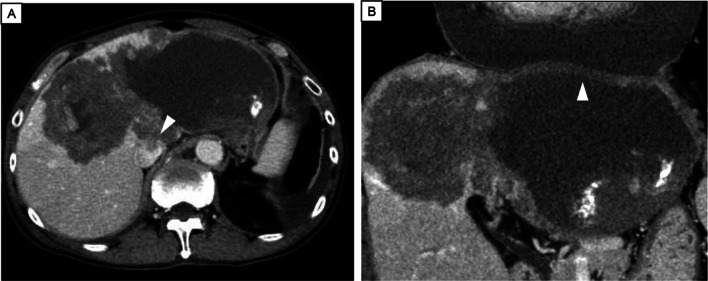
A CT showed a hepatic lesion with calcification. White arrow head: the echinococcal invasion of the IVC. B Hepatic lesion and pericardial fluid. White arrow head: echinococcal invasion of the pericardium
